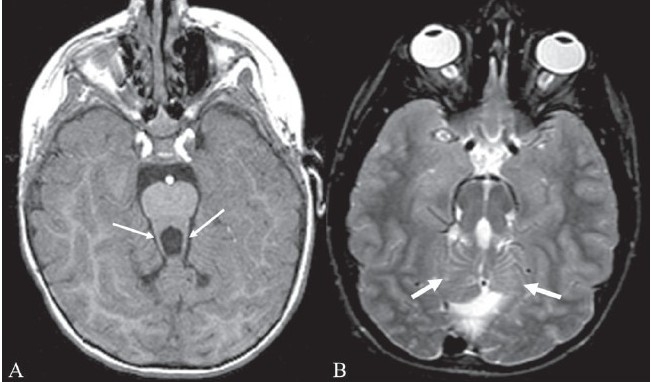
Molar tooth sign. Axial T1W MRI image of the brain (A) in a child with Joubert syndrome shows elongated superior cerebellar peduncles (arrows) giving the midbrain and superior peduncles the appearance of a molar tooth. Axial T2W image of the brain (B) in another child shows the molar tooth appearance of the midbrain and superior cerebellar peduncles. There is also hypoplasia of the cerebellar vermis (arrows)
